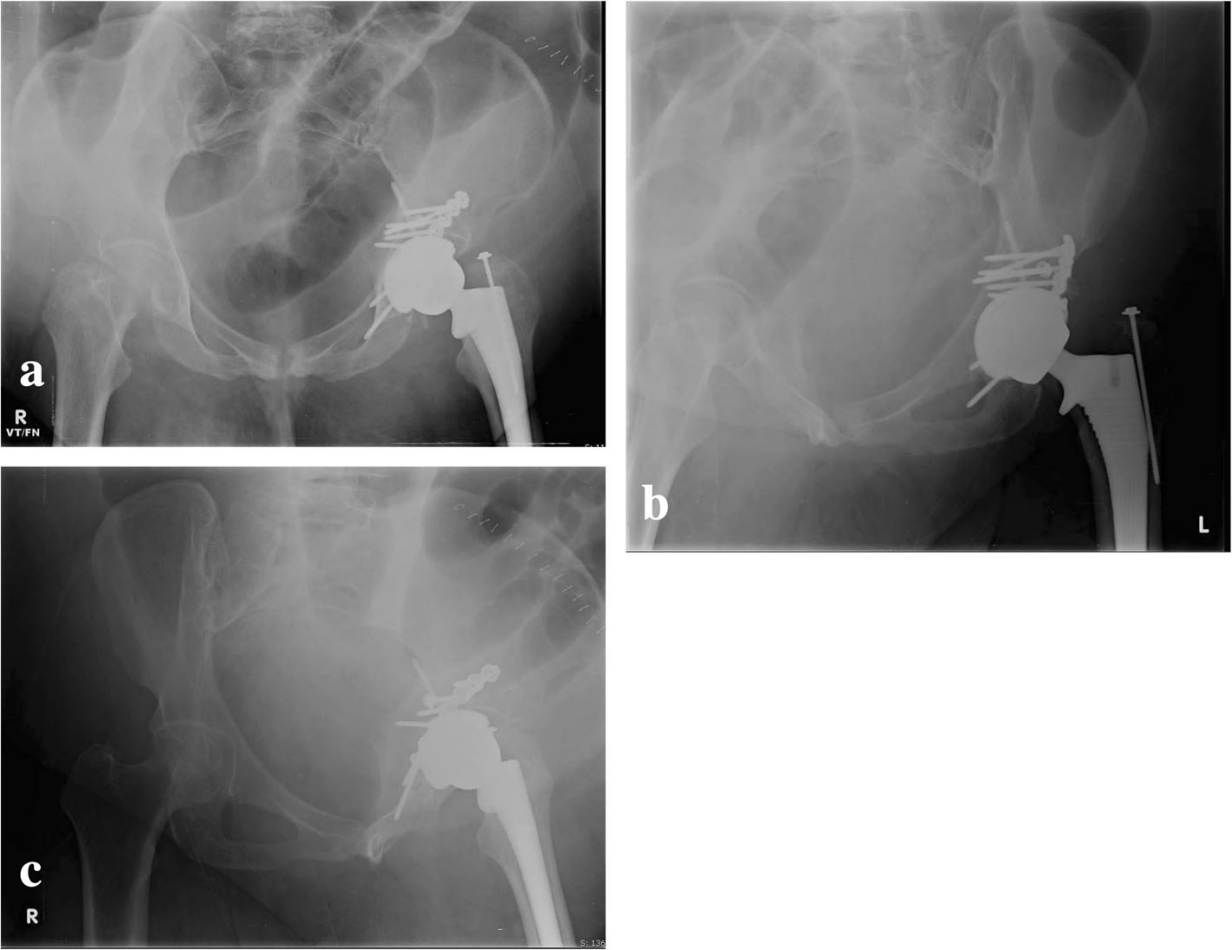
Post-operative AP pelvis (a) and Judet view (b–c) radiographs of a transtectal + posterior wall acetabular fracture after limited open reduction internal fixation and acute total hip arthroplasty
